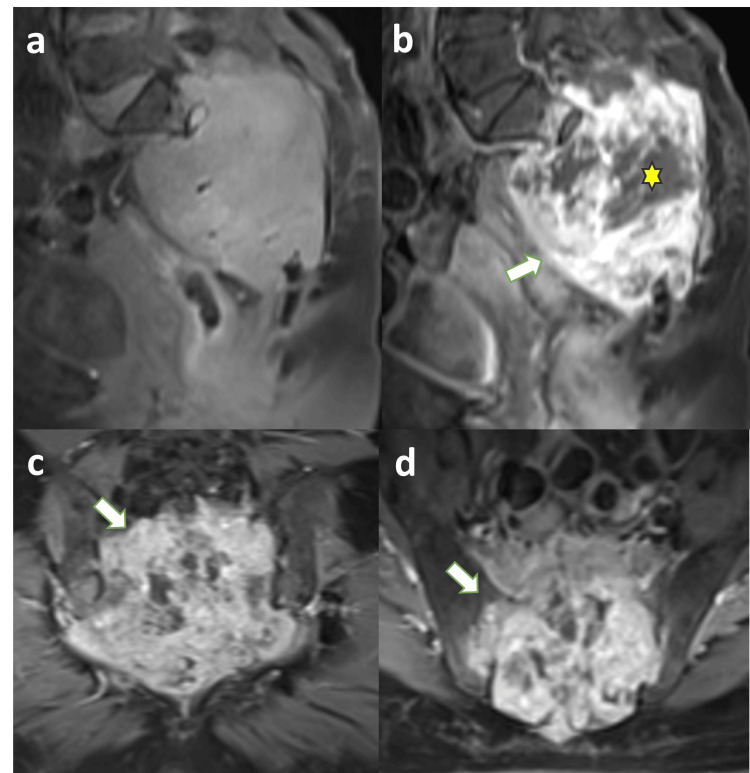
Contrast-enhanced MRI showing heterogeneous tumor enhancement Pre-contrast (a) and post-contrast fat-suppressed T1-weighted MRI images (b-d) demonstrate avid heterogeneous enhancement of the sacral soft tissue mass (arrows). Central non-enhancing regions (asterisk) correspond to necrotic or myxoid components within the lesion.
